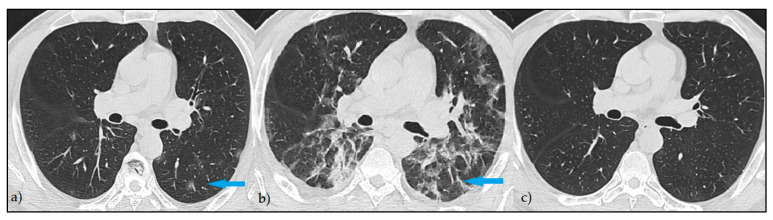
HRCT pattern of complete remission—Axial HRCT images: (a) minimal focal areas of GGO at the onset of the symptoms (blue arrow); (b) consolidation with a reticular pattern at 14 days from diagnostic (blue arrow); (c) complete remission of the lesions at 3 months.
